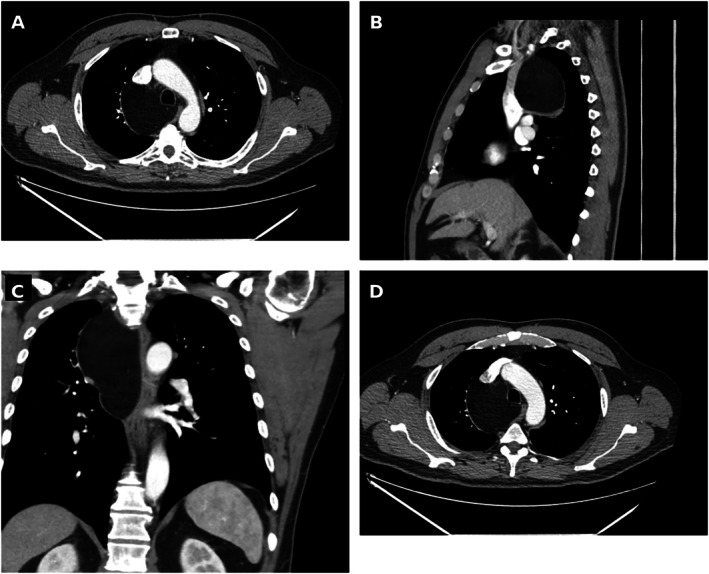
CT chest imaging demonstrating a giant anterior mediastinal lipoma. (A) Axial contrast‐enhanced CT at the level of the aortic arch showing a large anterior mediastinal mass with homogeneous fat attenuation (−100 HU), well‐defined smooth borders and no contrast enhancement. (B) Sagittal reconstruction demonstrating the full 13 cm craniocaudal extent of the mass from the thoracic inlet to the cardiac level. (C) Coronal reconstruction confirming the mass occupies the anterior mediastinum, displacing adjacent structures without invasion. (D) Axial CT at a more inferior level confirming homogeneous fat attenuation; the mass abuts the ascending aorta and main pulmonary artery without evidence of infiltration.
